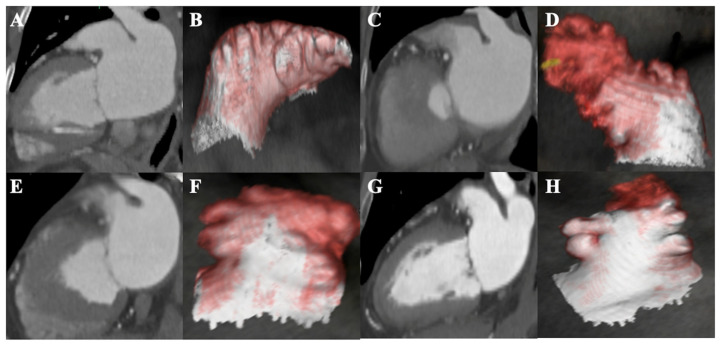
Left atrial appendage (LAA) morphology based on cardiac computed tomography (CT). Representative images of LAA morphologies from contrast-enhanced cardiac CT: axial views (A,C,E,G) and 3D volume-rendered reconstructions (B,D,F,H). (A,B) Chicken wing: Dominant lobe with a sharp bend near the proximal or mid segment. (C,D) Windsock: Single elongated dominant lobe with smooth curvature, lacking a sharp bend. (E,F) Cauliflower: Short, broad-based structure with no dominant lobe and multiple complex lobes near the ostium. (G,H) Cactus: Central dominant lobe with multiple secondary lobes projecting in superior and inferior directions, forming a forked appearance.
